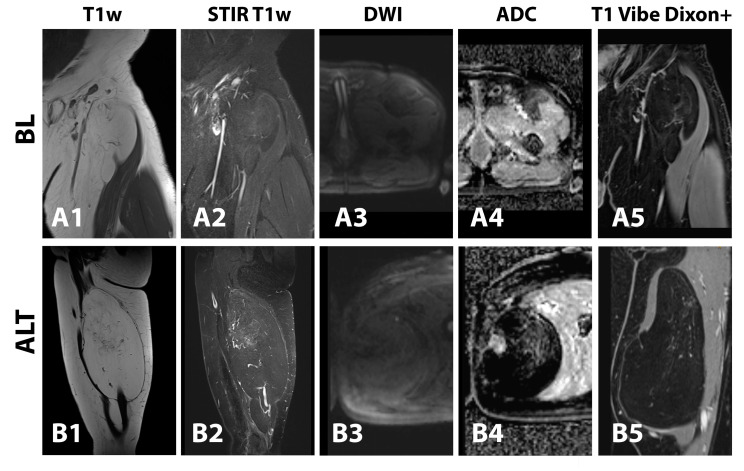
Examples of a benign (BL, (upper row)) and an atypical lipomatous tumor (ALT, (lower row)) in T1-weighted (1st column), short-tau inversion recovery T1-weighted (2nd column), diffusion-weighted (3rd column) images, ADC maps (4th column) and T1 VIBE Dixon images after intravenous contrast administration (5th column). Both tumors are predominantly composed of fat, yet the larger ALT shows intratumoral areas of patchy T1w signal decrease, STIR signal increase, atypical septation and patchy contrast enhancement.
